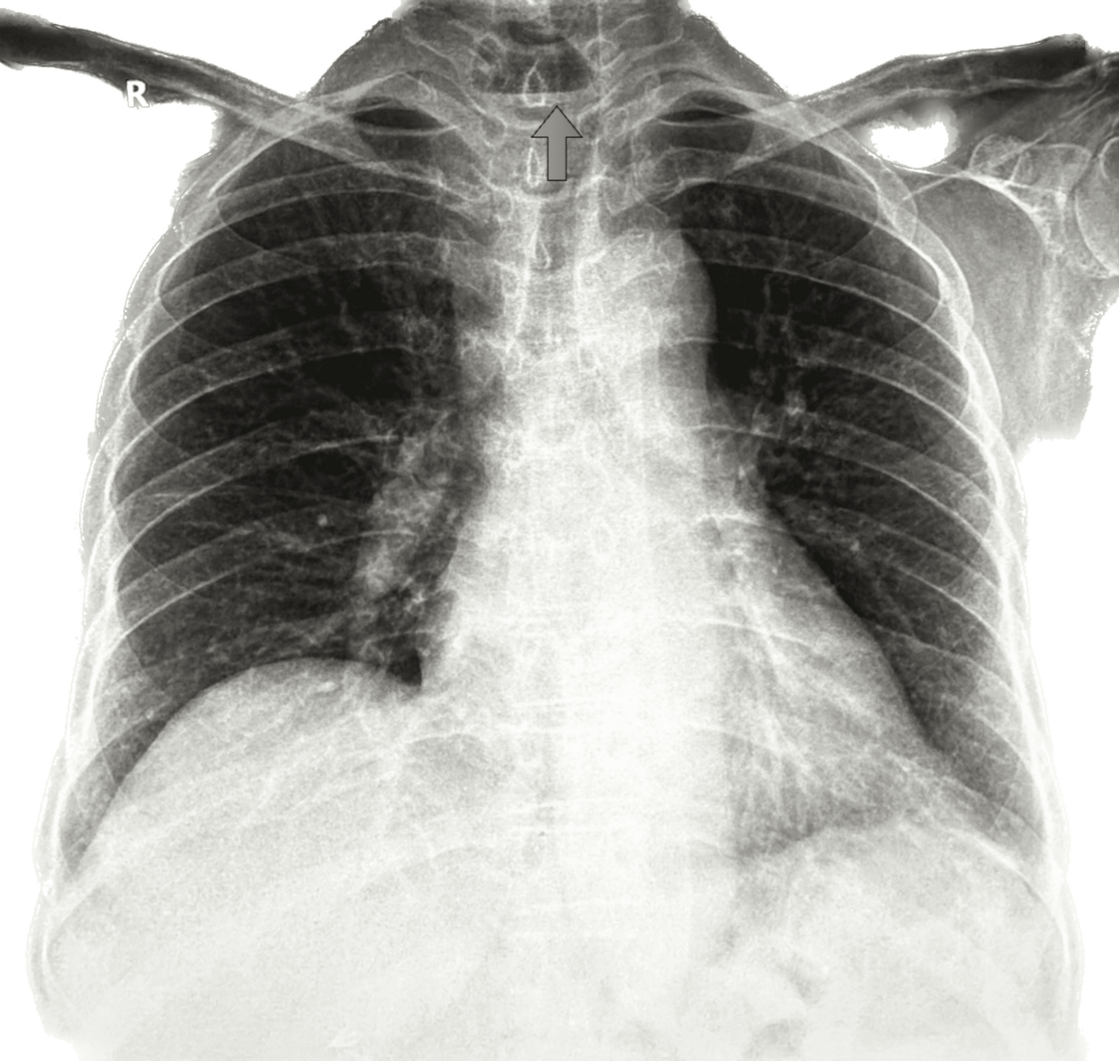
X-ray of the chest and cervical spine Showed the prevertebral post-tracheal space is widened in this current study, measuring 3.1 cm, with a lucent area that is seen in previous X-rays tracing back to 2008 with no air-fluid level present.
